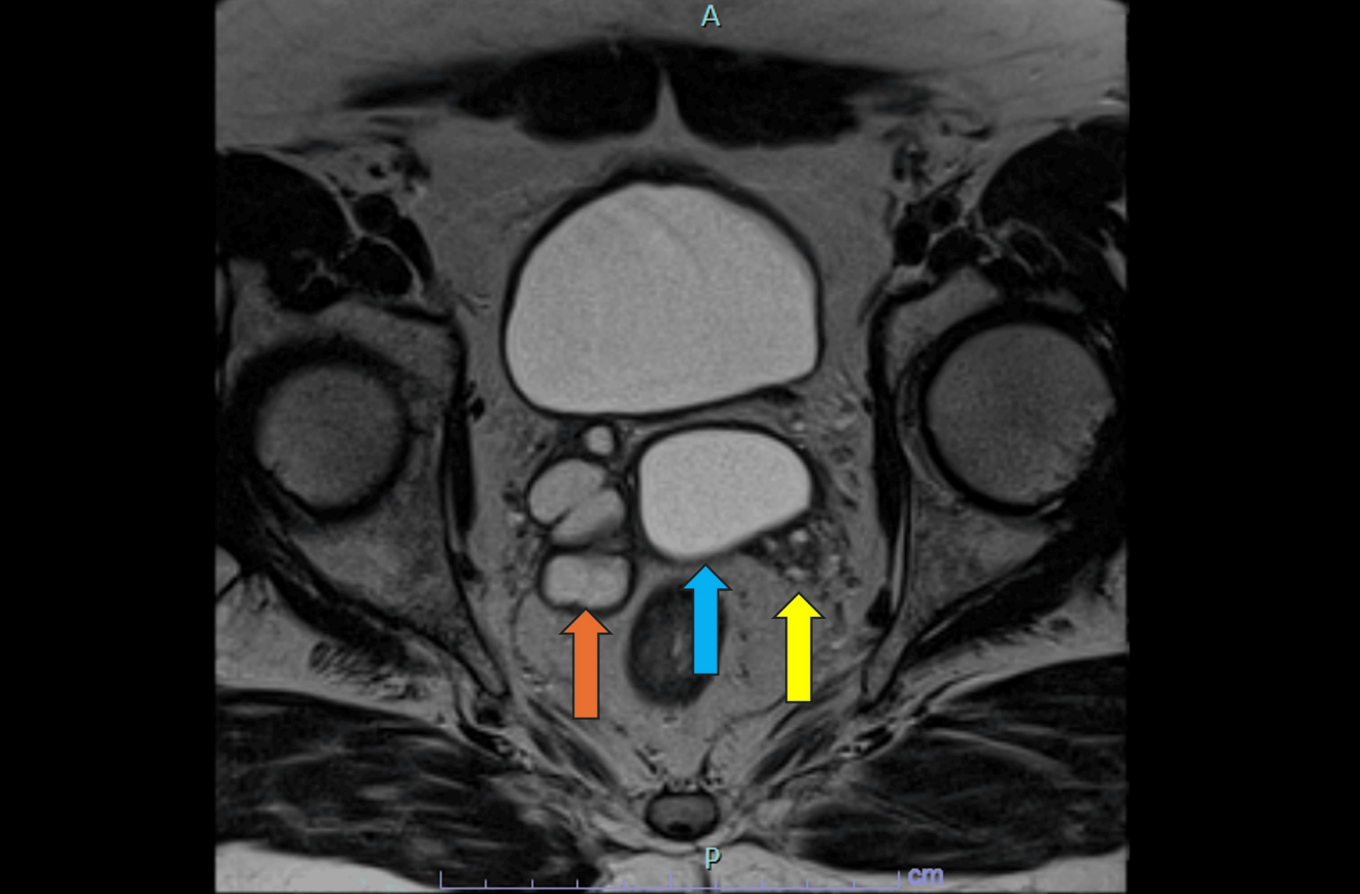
Axial T2-weighted (T2W) MRI of the pelvis showing the ejaculatory duct cyst (blue arrow) and the cystic dilation of the right seminal vesicle (orange arrow). The left seminal vesicle is indicated by the yellow arrow. The cystic right seminal vesicle is ipsilateral to the renal agenesis (see Figure 1), emphasizing the typical triad of Zinner syndrome. MRI: magnetic resonance imaging
